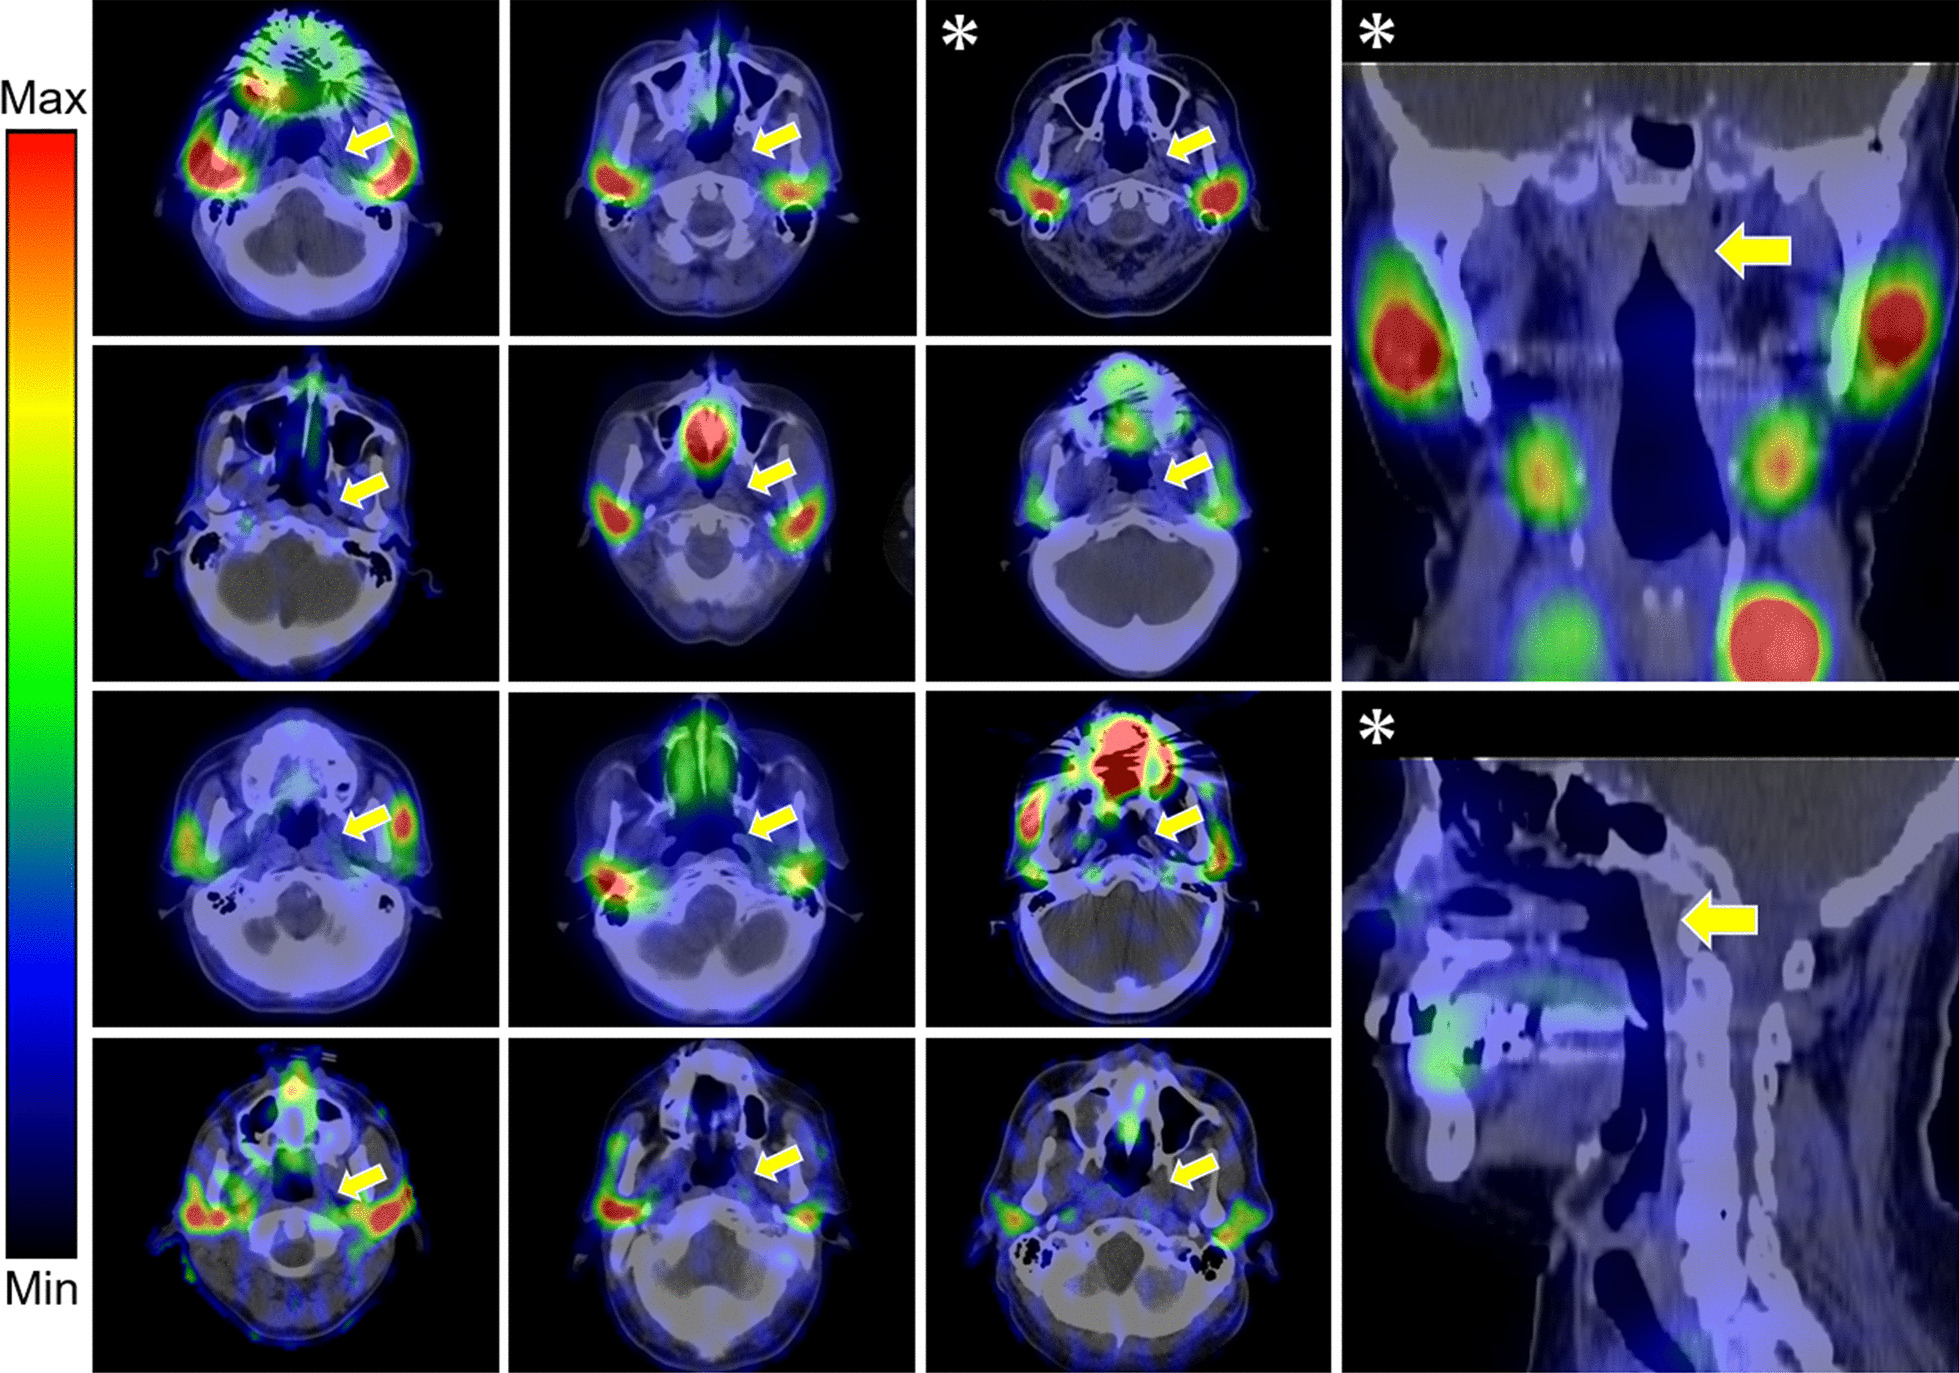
[^99m^Tc]pertechnetate SPECT/CT images of all the patients. Yellow arrows show the torus tubarius. Images marked with asterisks belong to the same patient
